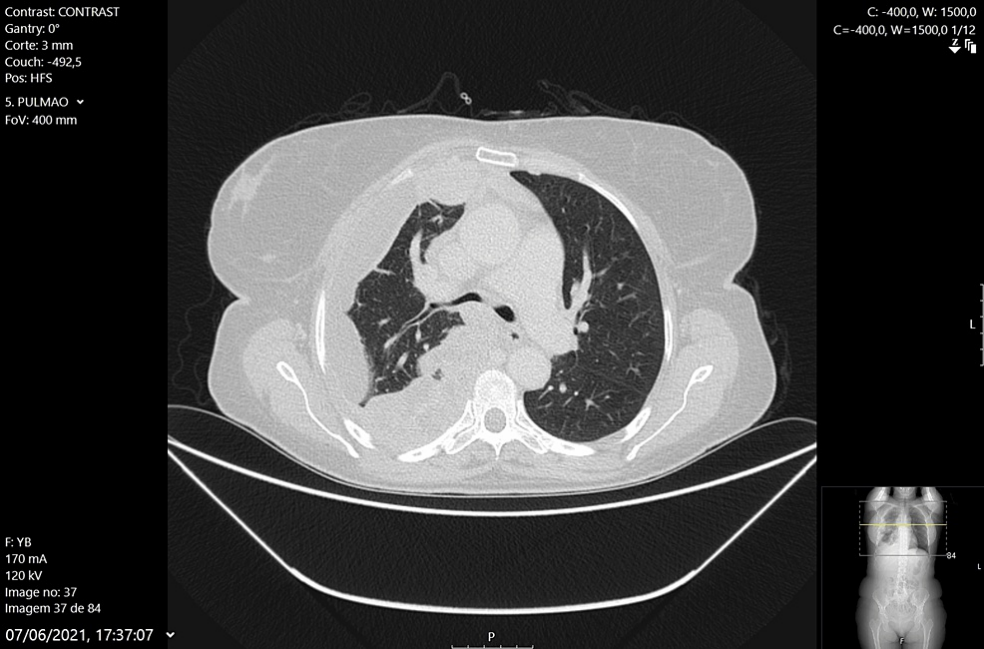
Thoracic CT scan during hospitalization showing rapid progression
